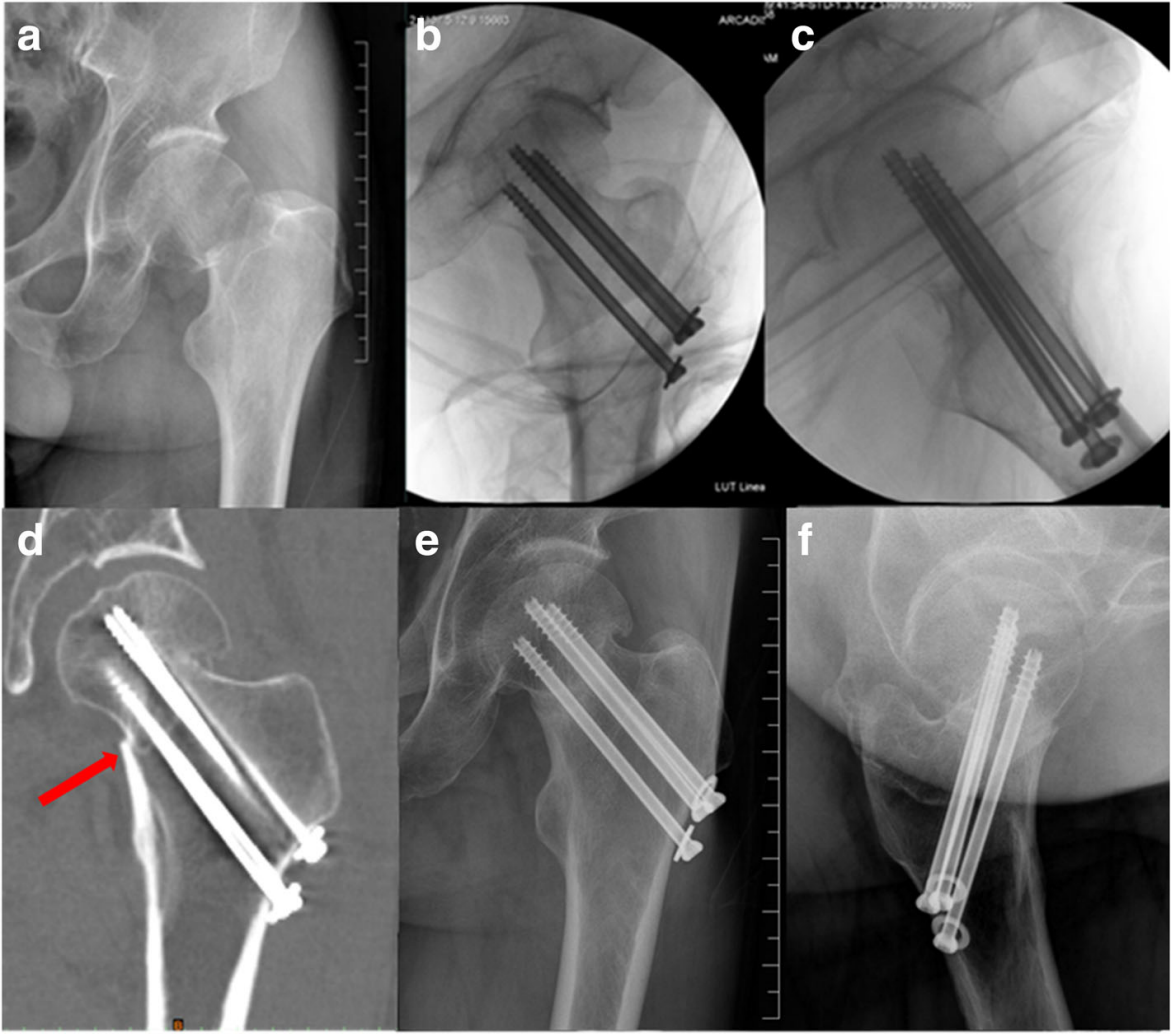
(a) A 56-year-old male suffered from displaced femoral neck fracture. (b, c) Intraoperative fluoroscopies showed that inferior cortical buttress pattern reduction was obtained. (d) CT scanning demonstrated the inferior medial cortices were resisted on a positive position (red arrow). (e, f) In six months follow-up, the fracture healed
